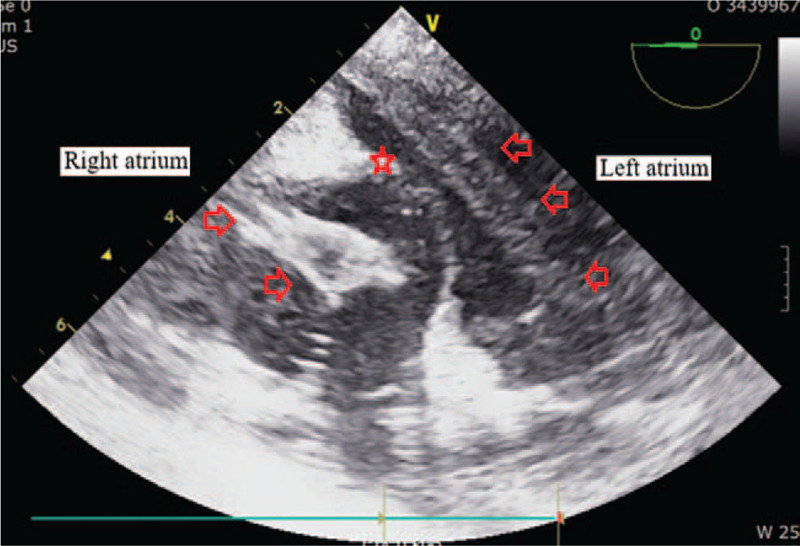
Multiple, huge thromboembolic clots in the right and left atria, seen on transesophageal echocardiography. Arrows point to the thromboembolic clots, and the star indicates the left-shifted atrial wall.
